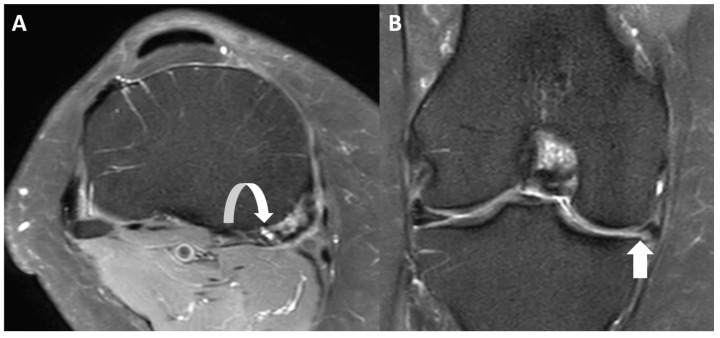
MRI scan of the right knee of a 40-year-old male patient who presented with medial-sided knee pain and knee locking. (A) Axial Proton Density Fat Saturated MRI image demonstating a focal fluid-filled defect at the insertion of the semimembranosus tendon in keeping with a focal tear (curved white arrow). (B) Coronal Proton Density Fat Saturated MRI image demonstrating undersurface tear of the body of the medial meniscus (white arrow).
